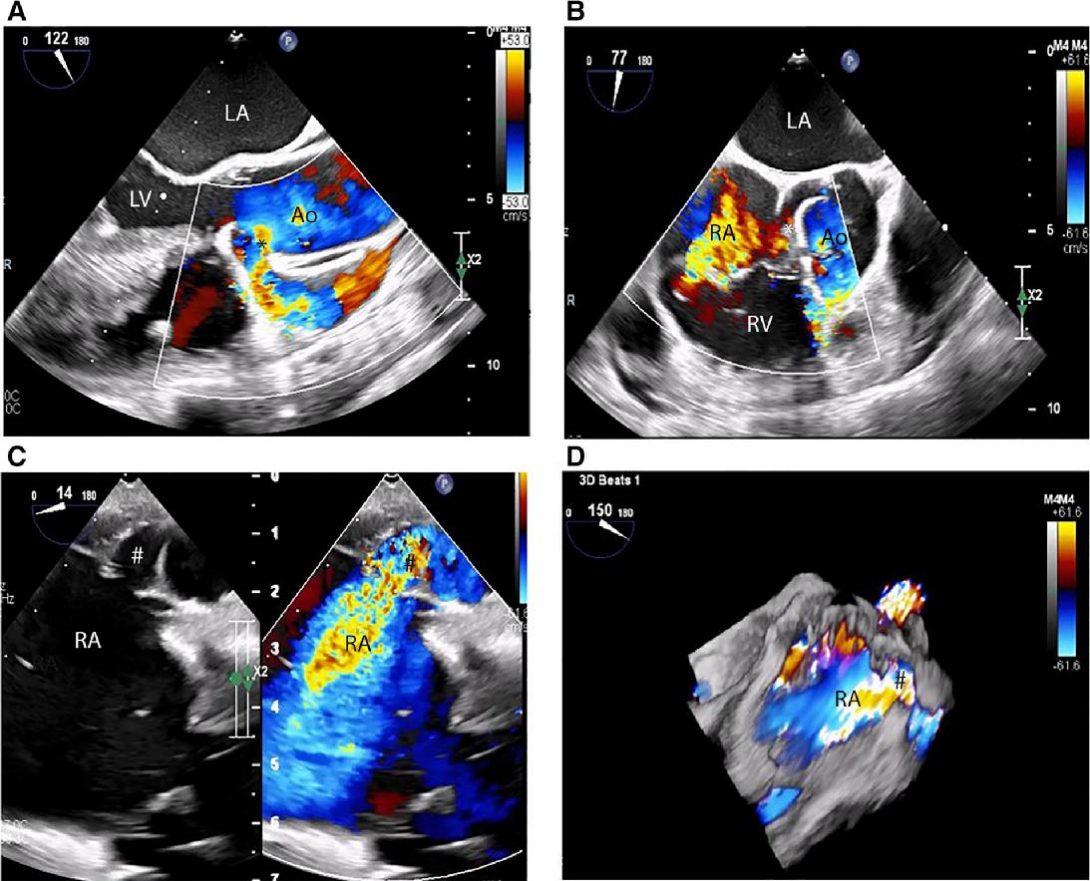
Intraoperative transoesophageal images acquired. (*A*) Mid-oesophageal long axis image demonstrating the origin and turbulent flow across the aneurysmal RCA (marked as asterisk). (*B*) Mid-oesophageal short axis image capturing both the origin of the RCA and severe jet of tricuspid regurgitation. (*C* and *D*) 2D and 3D rendered mid-oesophageal images delineating the termination site of the RCA fistula into the right atrium (marked as number sign). RA, right atrium; LA, left atrium; Ao, ascending aorta; LV, left ventricle; RV, right ventricle.
